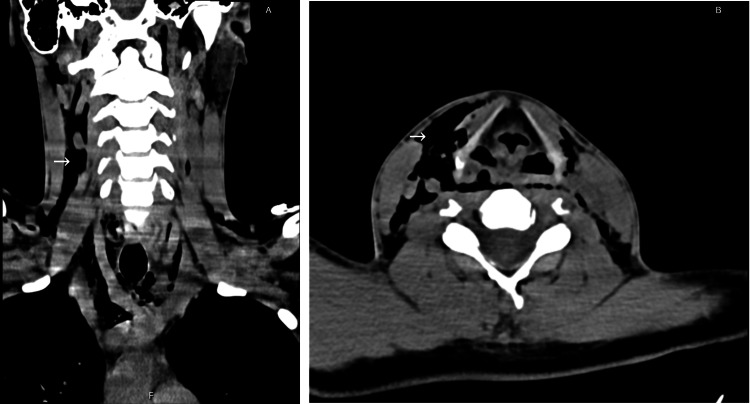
Cervical and subcutaneous emphysema (arrow) found in coronal computerized tomography (CT) scan (A) and transverse CT scan (B).
